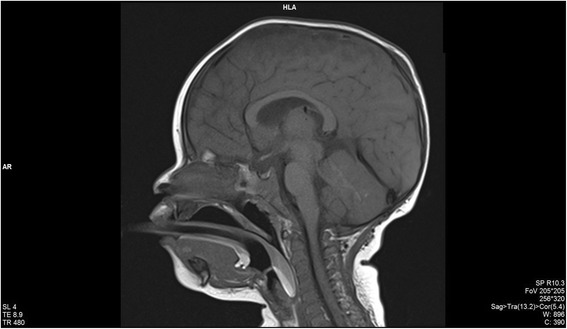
T1 MRI sequence, sagittal plane
